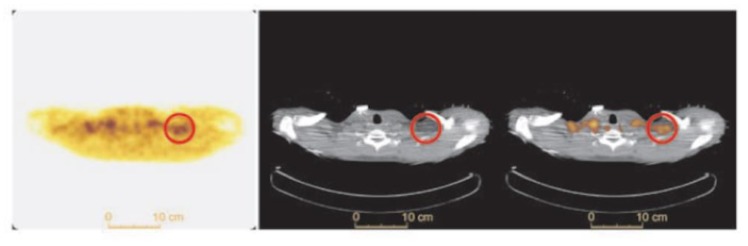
BAT FTHA uptake in a healthy human subject. Transverse FTHA-PET (left panel), CT (middle panel) and fused PET/CT (right panel) in a healthy subject after 1 h of cold exposure. Supraclavicular BAT is shown in the red circle. Image reproduced with permission from reference 28.
